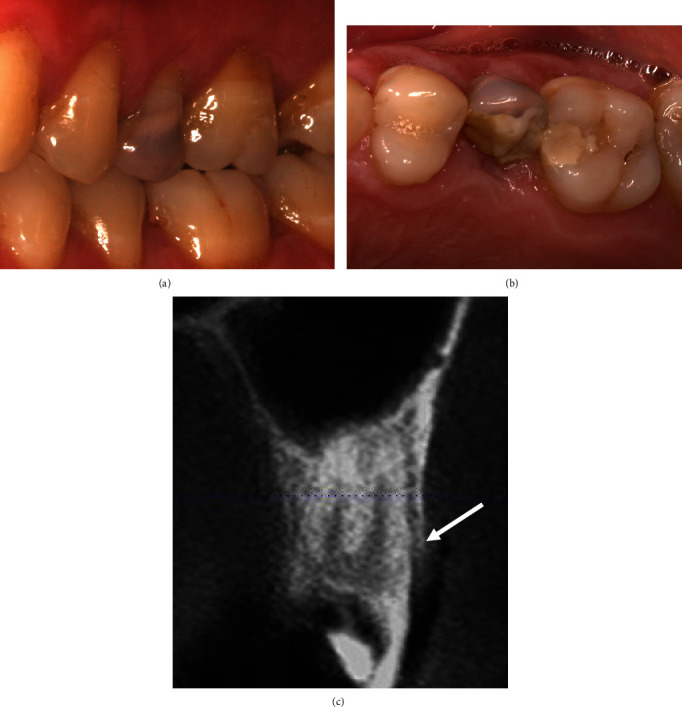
(a) The buccal and (b) occlusal views showed the maxillary left second premolar (tooth #25) was heavily filled and unrestorable, and therefore, indicated for extraction. (c) Baseline cross-sectional CBCT scan showed buccal dehiscence associated with the tooth #25 (the buccal alveolar crest is marked by arrow).
